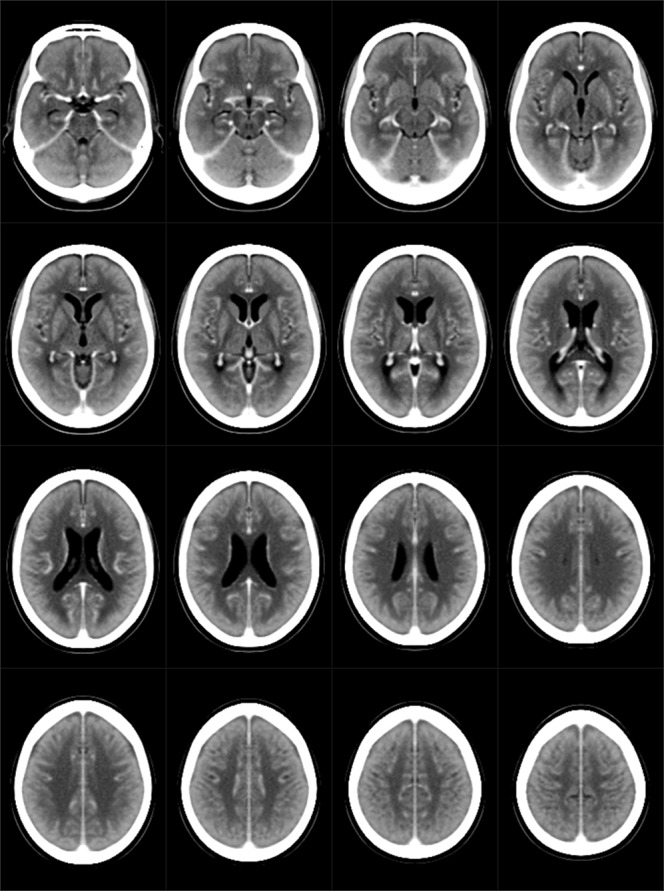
A CT template set for the topographic mapping. The original stroke-control CT template^11^, with the axial slicing angle being in line with the anterior-posterior commissure line, was realigned so that they become parallel to the orbito-meatal line.
